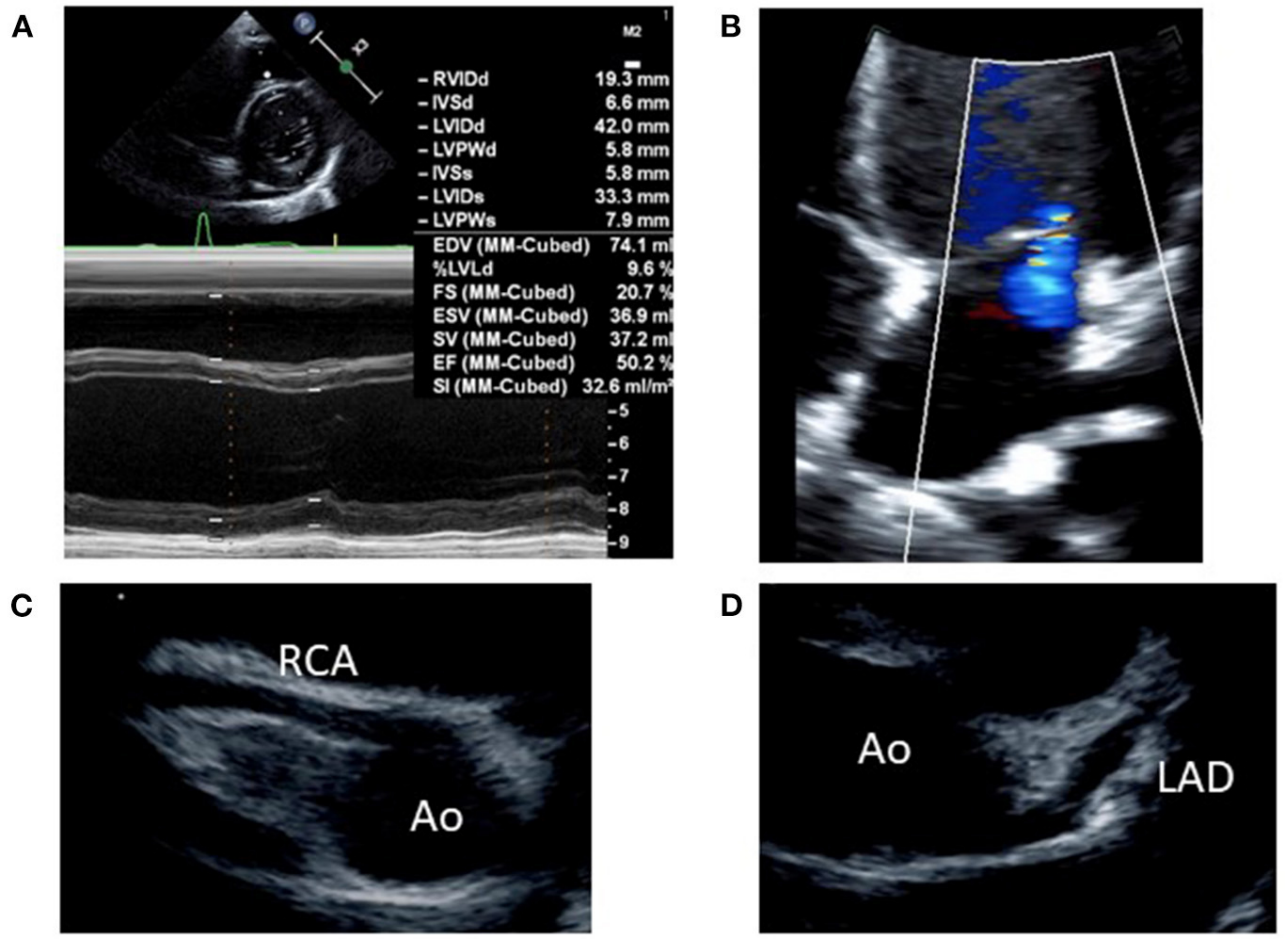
Echocardiography findings on the 6th day of illness: (A) M-mode (left ventricular ejection fraction, 50.2%), (B) mild mitral regurgitation by 4-chamber view, (C) right coronary artery (seg1: 3.0 mm, *Z* score+1.6), *Z* score was calculated by “*Z* score calculator of coronary arterial diameter” (http://raise.umin.jp/zsp/calculator/), (D) left coronary artery (seg5: 3.0 mm, *Z* score+0.9, seg6: 2.7 mm, *Z* score+1.3, seg11: 2.6 mm, *Z* score+1.5). Ao, aorta; RCA, right coronary artery; LAD, left anterior descending artery.
